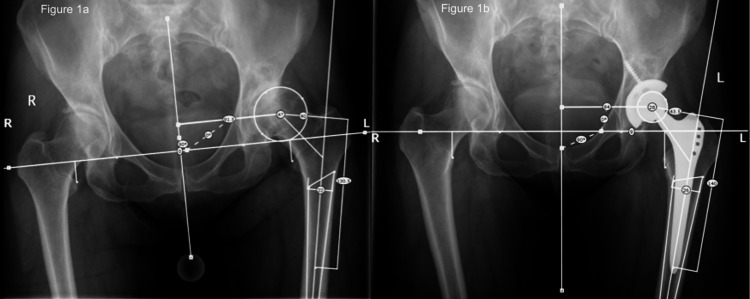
Pelvic radiographs depicting pre-operative (1a) and post-operative (1b) measurement of leg length and offset
